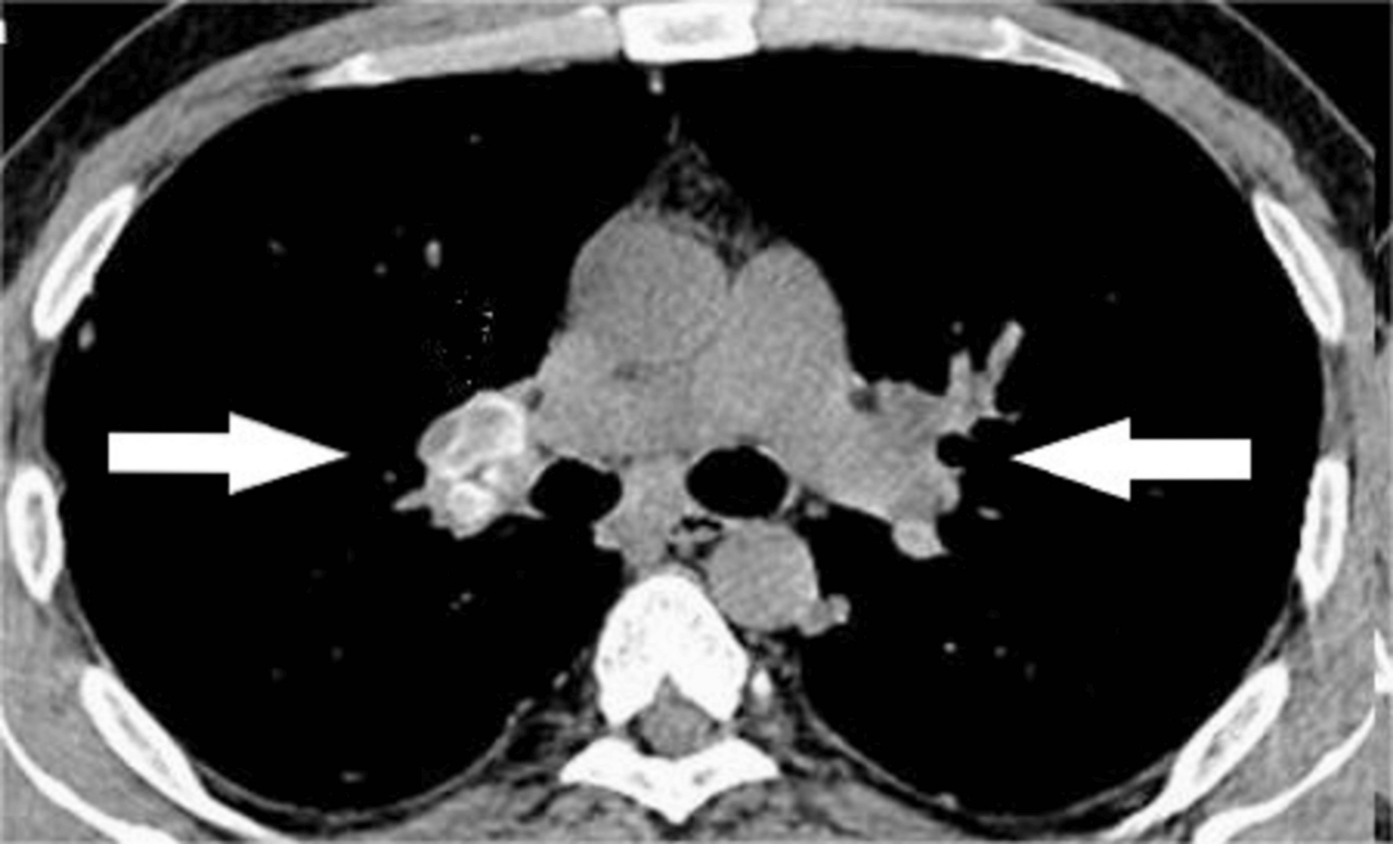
Chest computed tomography. Arrows show enlarged hilar lymph nodes.
